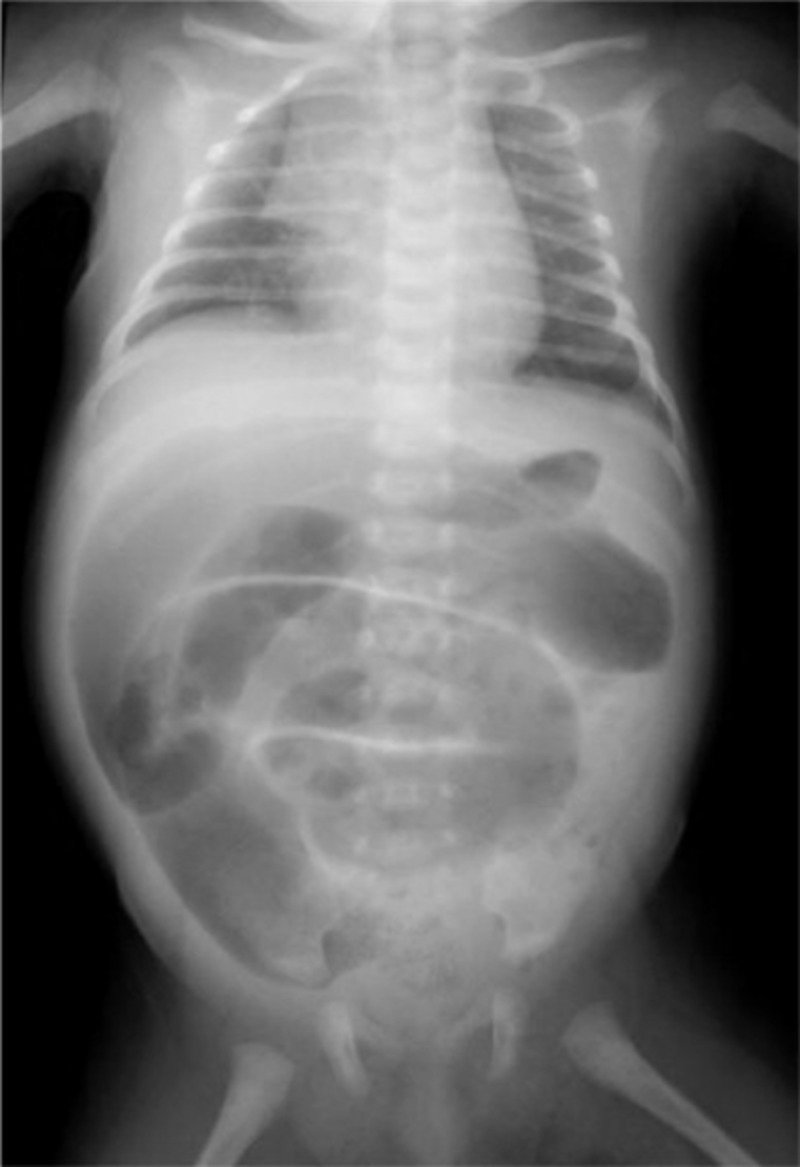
Abdominal plain X-ray shows a generalized distention of the loops of the large intestine. Typical findings of Hirschsprung disease were seen. (Consent was obtained to publish this radiograph).
